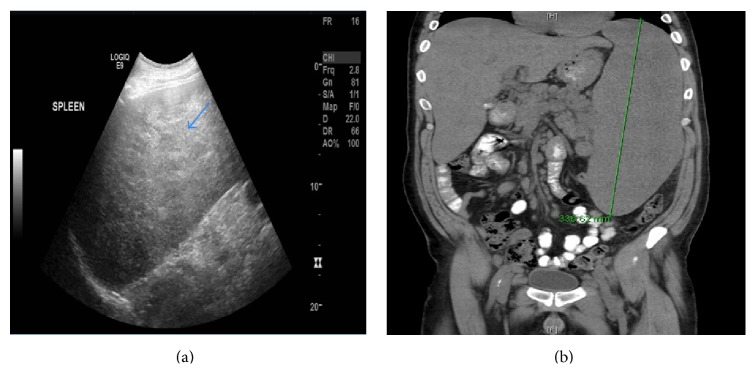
Massive splenomegaly. (a) Sagittal sonographic view of the spleen showing a markedly enlarged and diffuse heterogeneous spleen (blue arrow) measuring 30 cm in length. (b) Coronal noncontrast CT of the abdomen and pelvis showing enlarged spleen reaching 33.6 cm in length.
